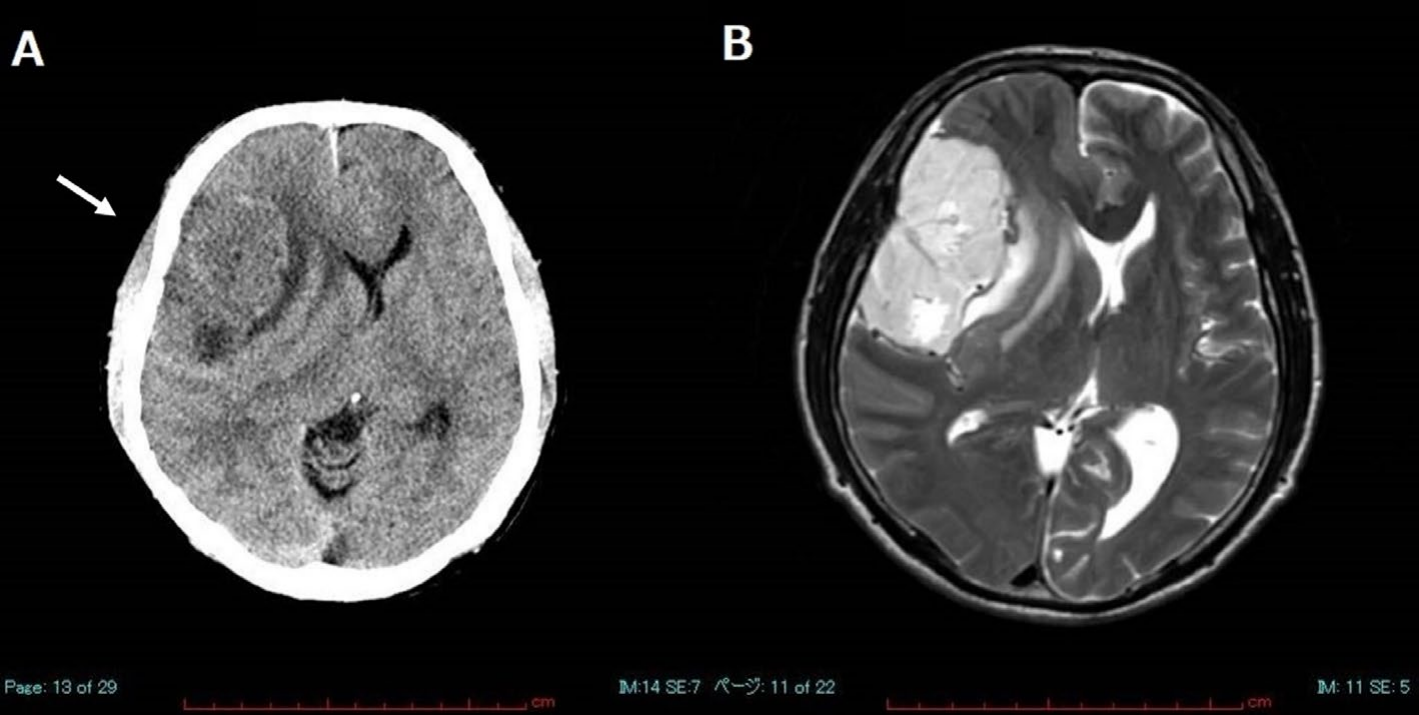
(A) Computed tomography showing an irregularly shaped, hypodense tumor (arrow) measuring approximately 6 cm in diameter in the right frontal dome extending to the sphenoid margin. (B) T2‐weighted magnetic resonance imaging showing high signal intensity.
